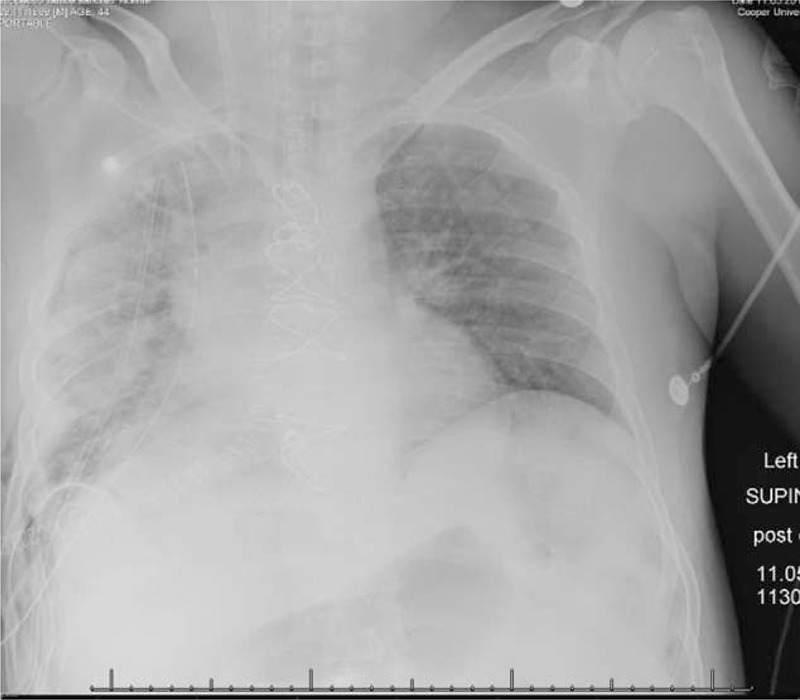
Postoperative chest x-ray with reexpansion of a large portion of the right lung.
